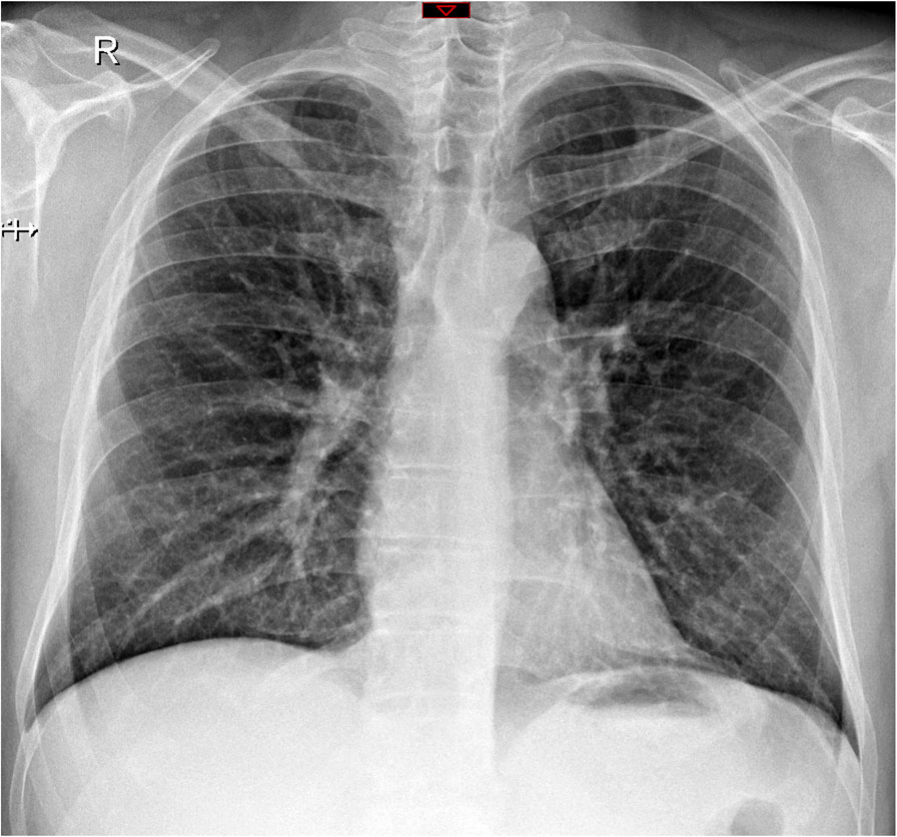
Sixty-five-year-old woman without comorbidities with H1N1 infection who did not have a worse outcome. Normal chest radiography performed at emergency department
